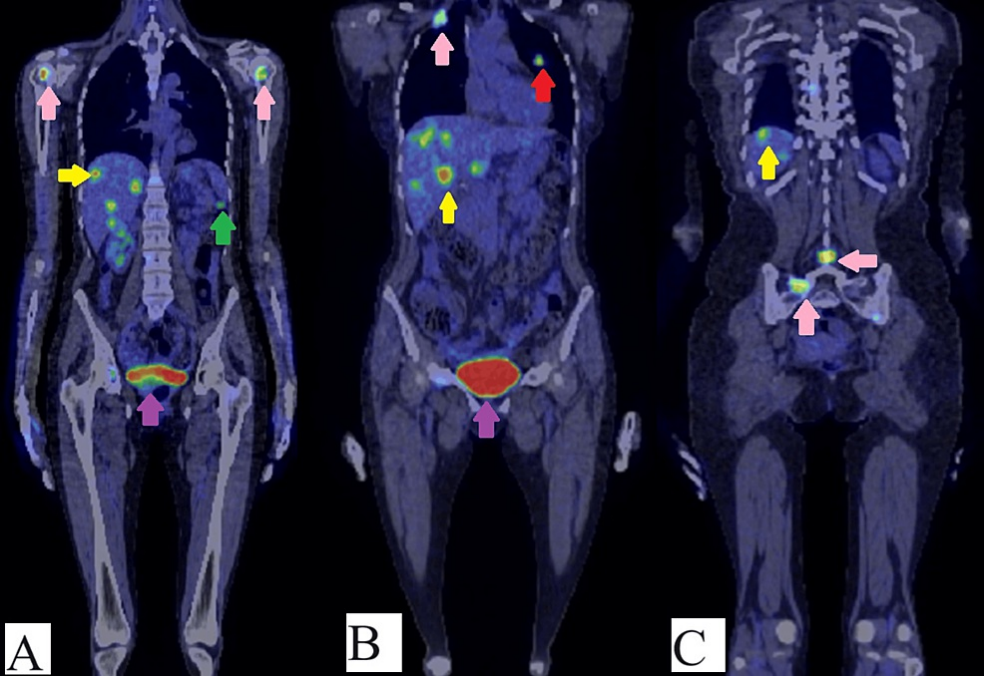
PET-CT images. (A) Multiple osteolytic lesions with an abnormal increase in metabolism on both heads of the humerus (pink arrow), in the left colic flexure an abnormal increase in metabolism (green arrow), abnormal increase metabolism in the liver - multiple metastases (yellow arrow) and urinary bladder, FDG accumulation (purple arrow); (B) abnormal increase metabolism: in the lung (red arrow), in liver (multiple metastases) (yellow arrow), first rib (osteolytic lesion) (pink arrow) and urinary bladder, FDG accumulation (purple arrow); (C) abnormal increase metabolism on pelvic bones and L4 spinous vertebra, process extended in the soft adjacent tissue (pink arrows) and liver metastases (yellow arrow).
